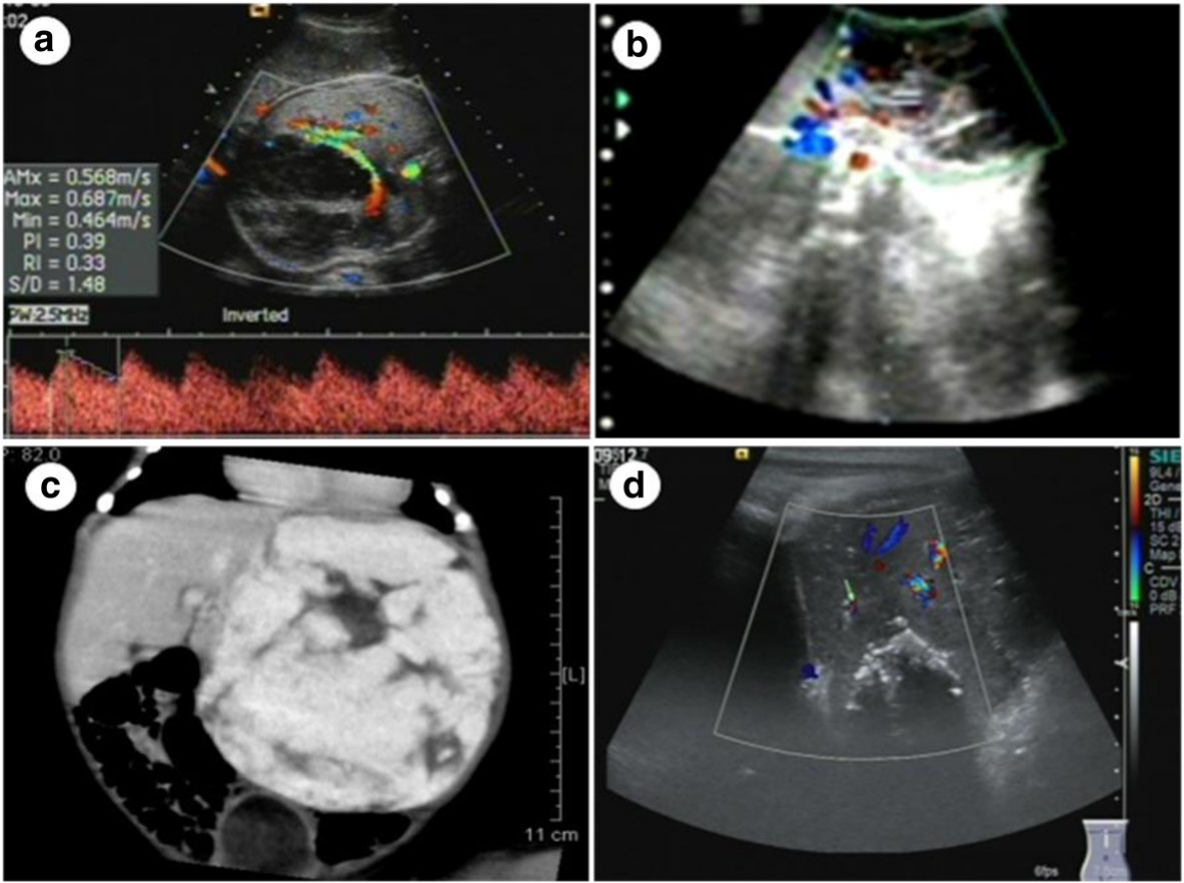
Fetus with a hemangioma in the left liver lobe (fetus 1). a Circular hypoechoic lesion, measuring 86 × 81 × 103 mm. Color Doppler ultrasound shows a ring of blood flow at the edge of the lesion and stars intralesion. Pulse Doppler ultrasound suggests that the resistance index is 0.33. b Ultrasound on the day of birth shows that lesion has a clear border, an excentric growth pattern, and an internal grid-shaped region and bypasses the left hepatic vein. c Postnatal computed tomography (CT) shows the lesion in the left lobe. On contrast-enhanced CT, the lesion was centripetal enhancement. d Two years after treatment of the neonate with propranolol and dexamethasone, the lesion was 35 × 36 × 34 mm in size, with multiple strong echo spots in the center
